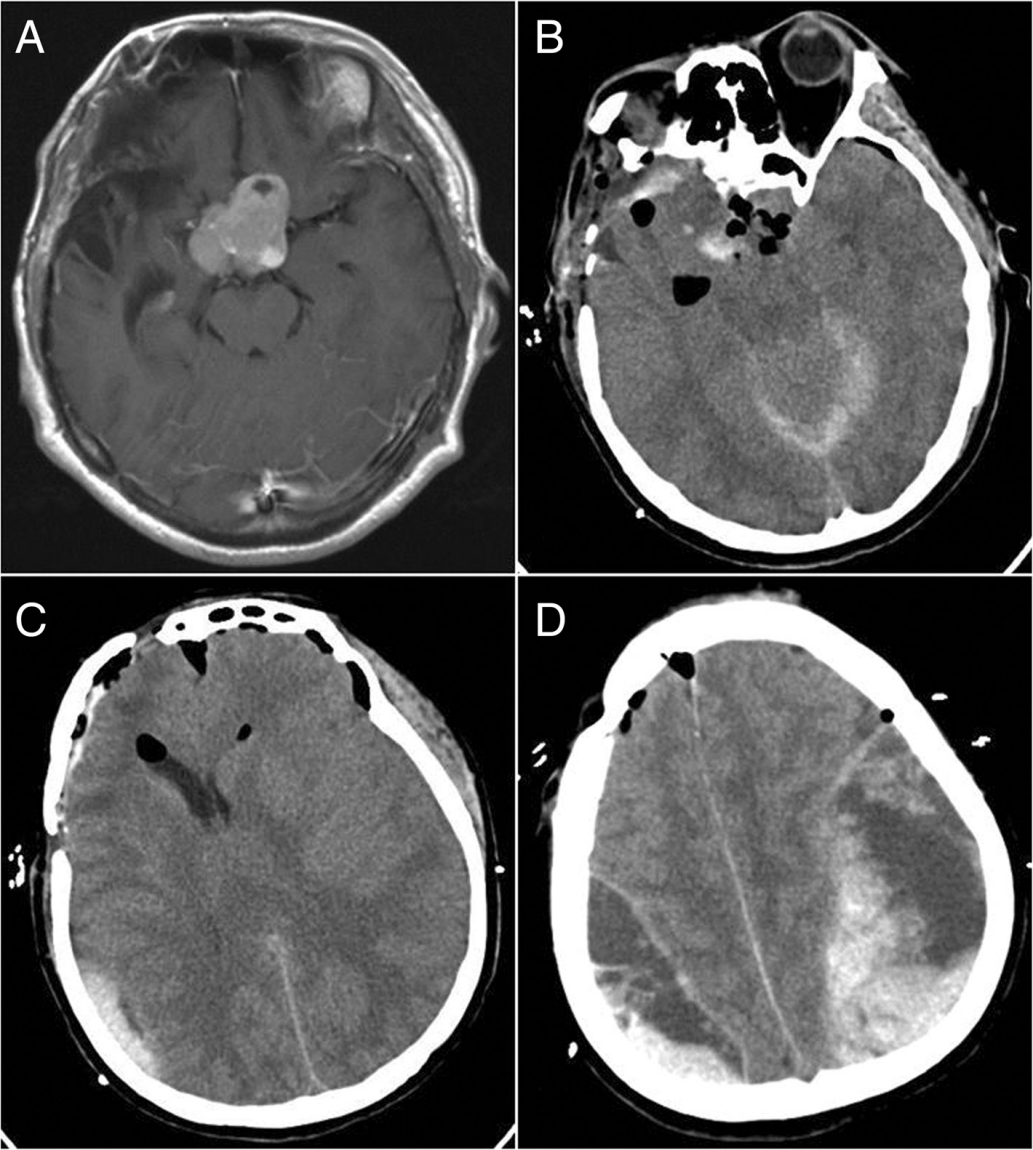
Bilateral remote hemorrhage in case 11. a Preoperative-enhanced MRI revealed a sellar tumor. b CT showed the tumor resection. c, d CT revealed epidural hematomas at the tops of bilateral sides
